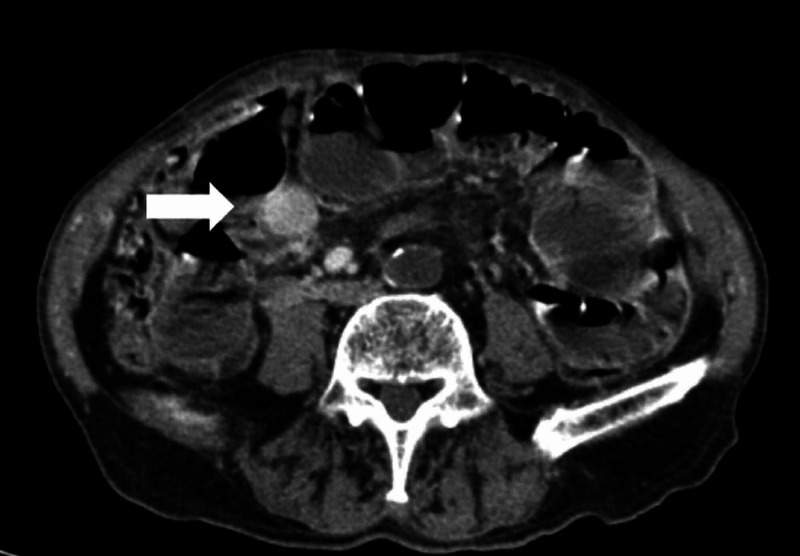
Abdomino-pelvic CT (axial section) revealing the gallstone (white arrow) impacted in terminal ileum, causing proximal small bowel dilatation.
